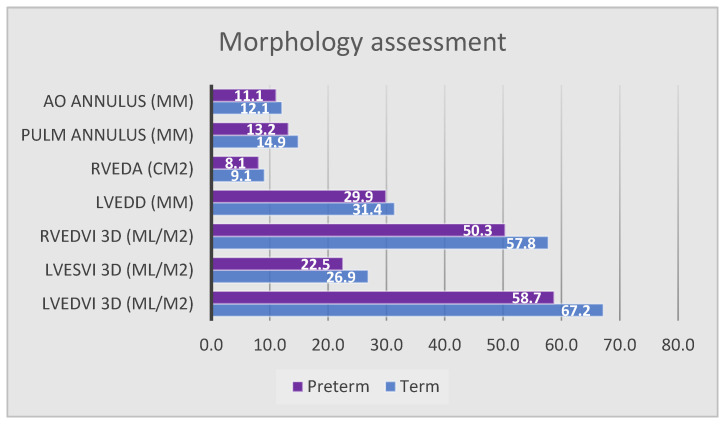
Echocardiographic morphologic parameters, significant results. Data reported as mean. LVEDD, left ventricle end-diastolic diameter; LVEDVi, indexed left ventricle end-diastolic volume; LVESDi, indexed left ventricle end-systolic diameter; LVESVi, indexed left ventricle end-systolic volume; RVEDA, right ventricle end-diastolic area; RVEDVi, indexed right ventricle end-diastolic volume.
